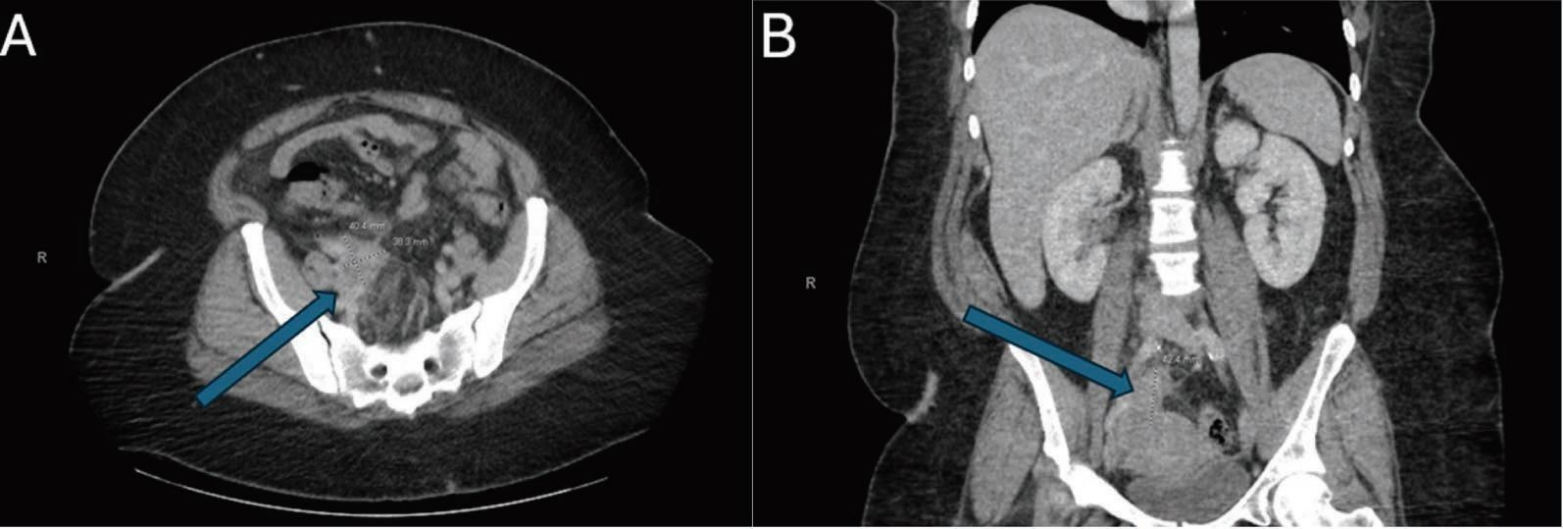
Computed tomography (CT) of the abdomen and pelvis with soft tissue thickening adjacent to the colon with (A) axial and (B) coronal slices from a CT abdomen pelvis with intravenous contrast demonstrating a 4 cm × 4 cm area of soft tissue thickening adjacent to the uterine fundus, colon, cecum, and terminal ileum concerning for pelvic inflammatory disease (blue arrows point to this area on both panels). A tubo‐ovarian abscess could not be excluded.
